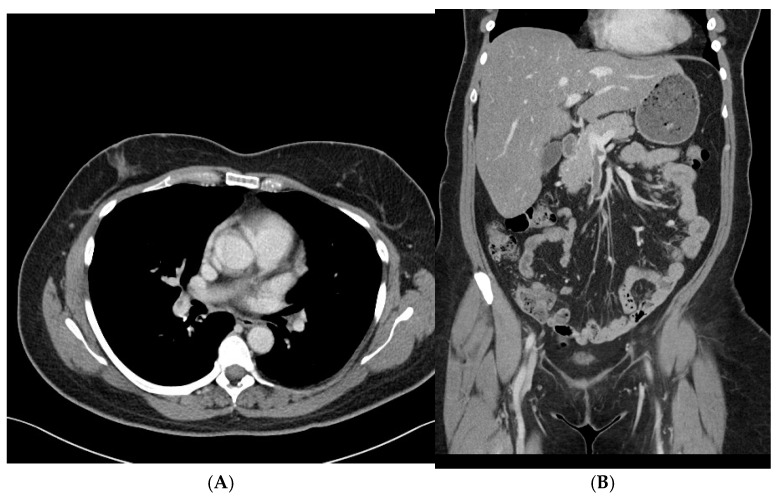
(A,B) Contrast-enhanced CT imaging shows non-occlusive thrombi in the portal and superior mesenteric veins, along with multiple non-occlusive emboli in the pulmonary arteries.
